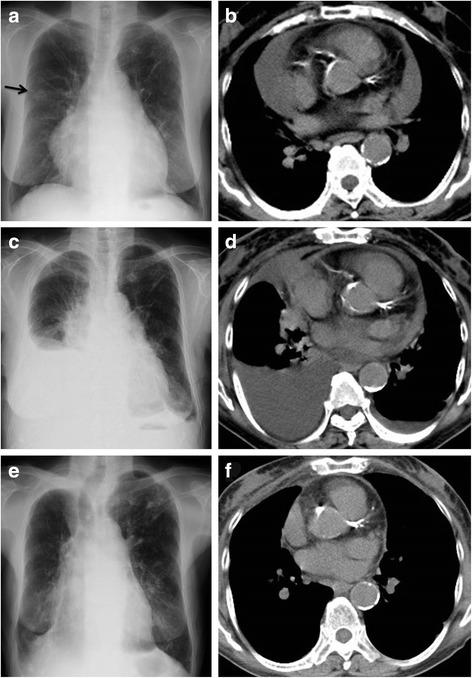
Representative photographs during the clinical course. Chest X-ray and computed tomography (CT) scan 4 months before admission, which was 2 months before the lung cancer operation, revealed cardiac enlargement with a nodular shadow (arrow) in the right lung field (a) and a large volume of pericardial effusion (b). On admission, a chest X-ray showed the right pleural effusion as well as blunting of the left costophrenic angle (c), and a chest CT scan showed that the pericardial effusion had decreased compared with that before the lung operation (d). No relapse of the pleural and pericardial effusion was confirmed by chest X-ray or a CT scan 8 months after removal of the fibroma (e, f)
